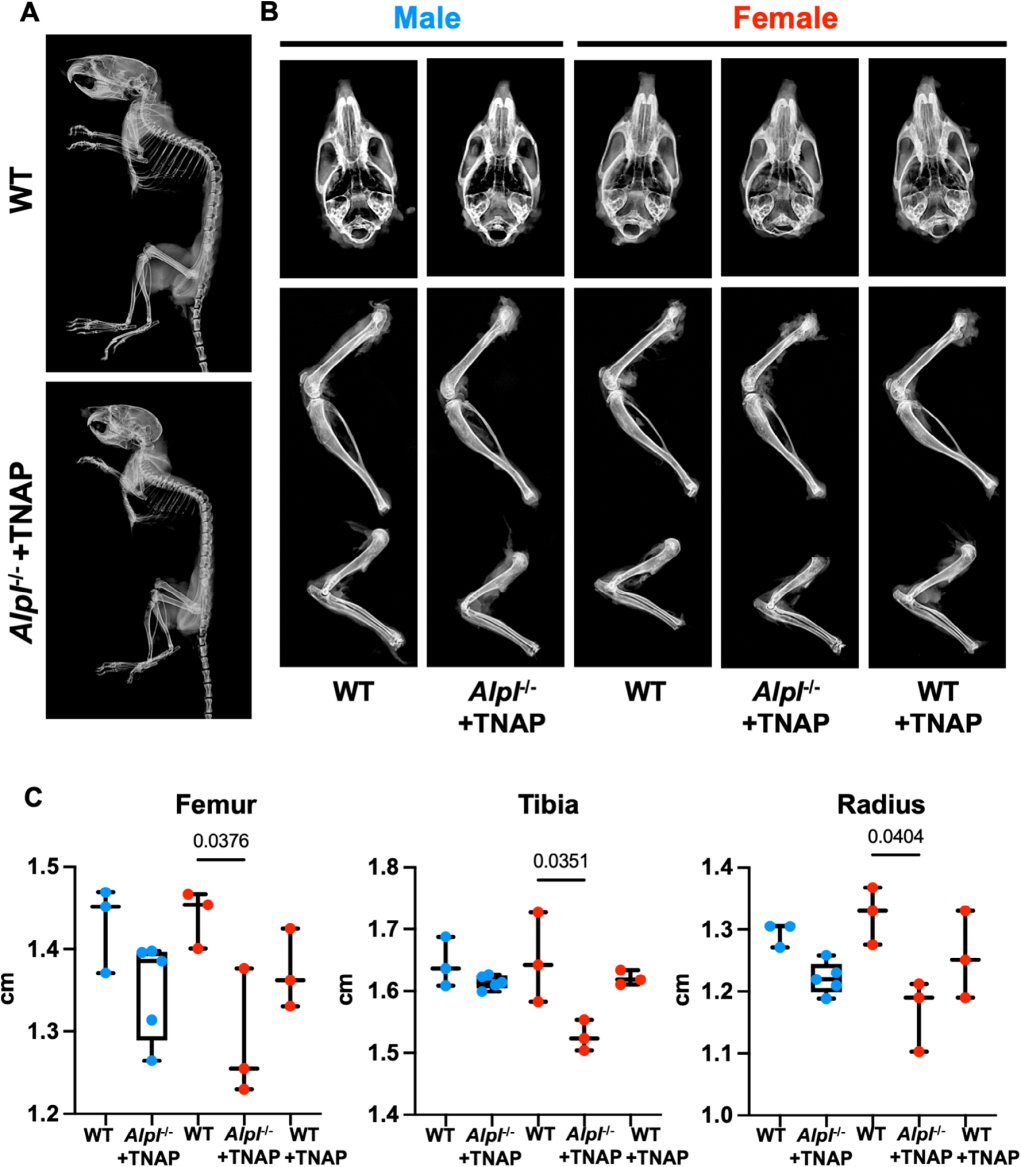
Improved radiographical findings in AAV8‐TNAP‐D_10_‐treated *Alpl*
